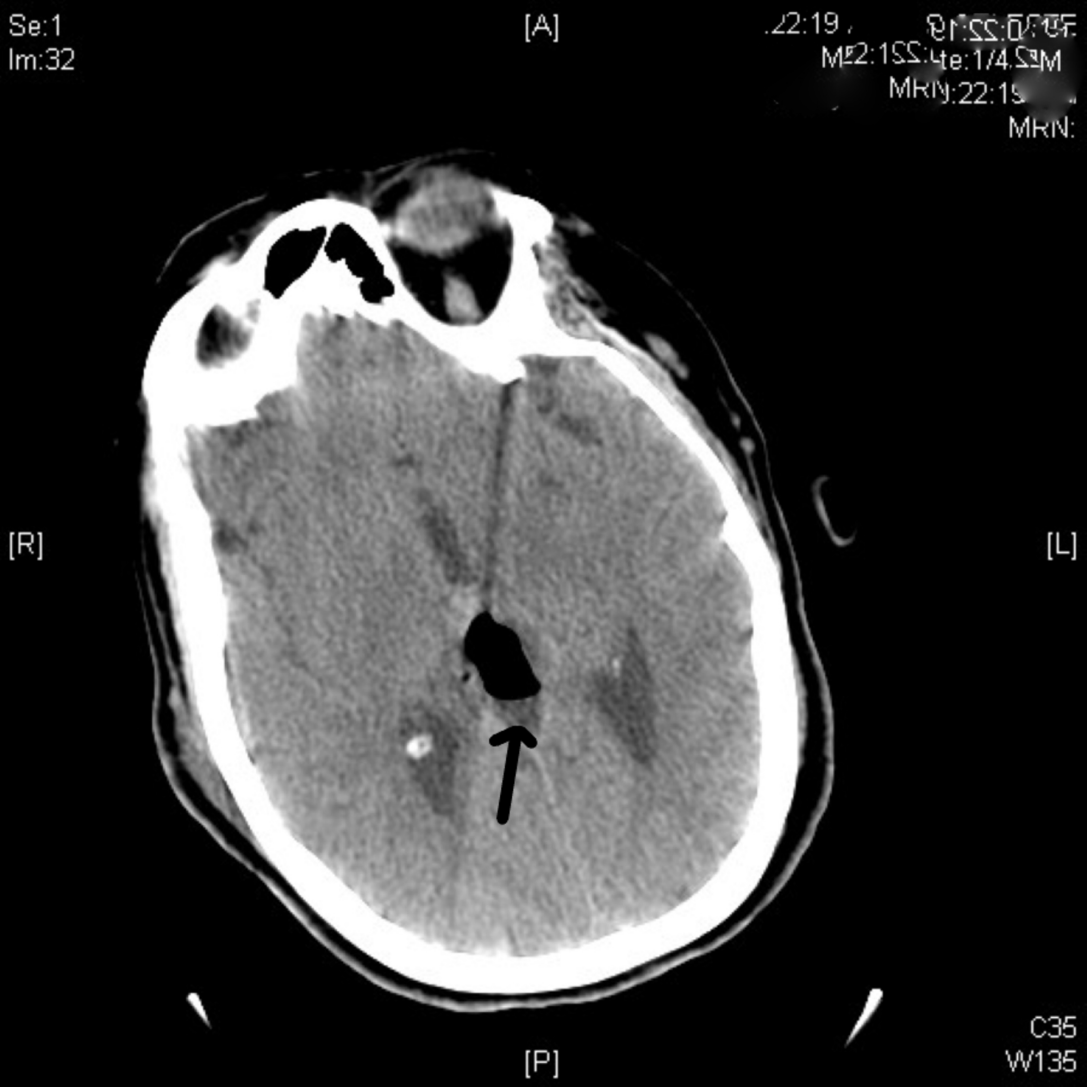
Axial CT scan showing postoperative pseudomeningocele CT: computed tomography
